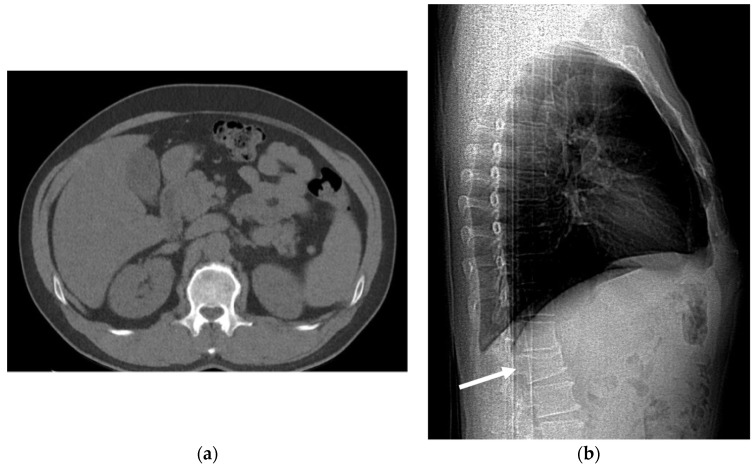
(a) An axial low-dose computed tomography (LDCT) scan of a 56-year-old male at the level of the first lumbar vertebra demonstrating no significant musculoskeletal abnormalities. (b) A lateral scout view of the same LDCT scan clearly revealing the compression fracture of the first lumbar vertebral body (arrow), highlighting the importance of a comprehensive scan review for accurate diagnosis.
